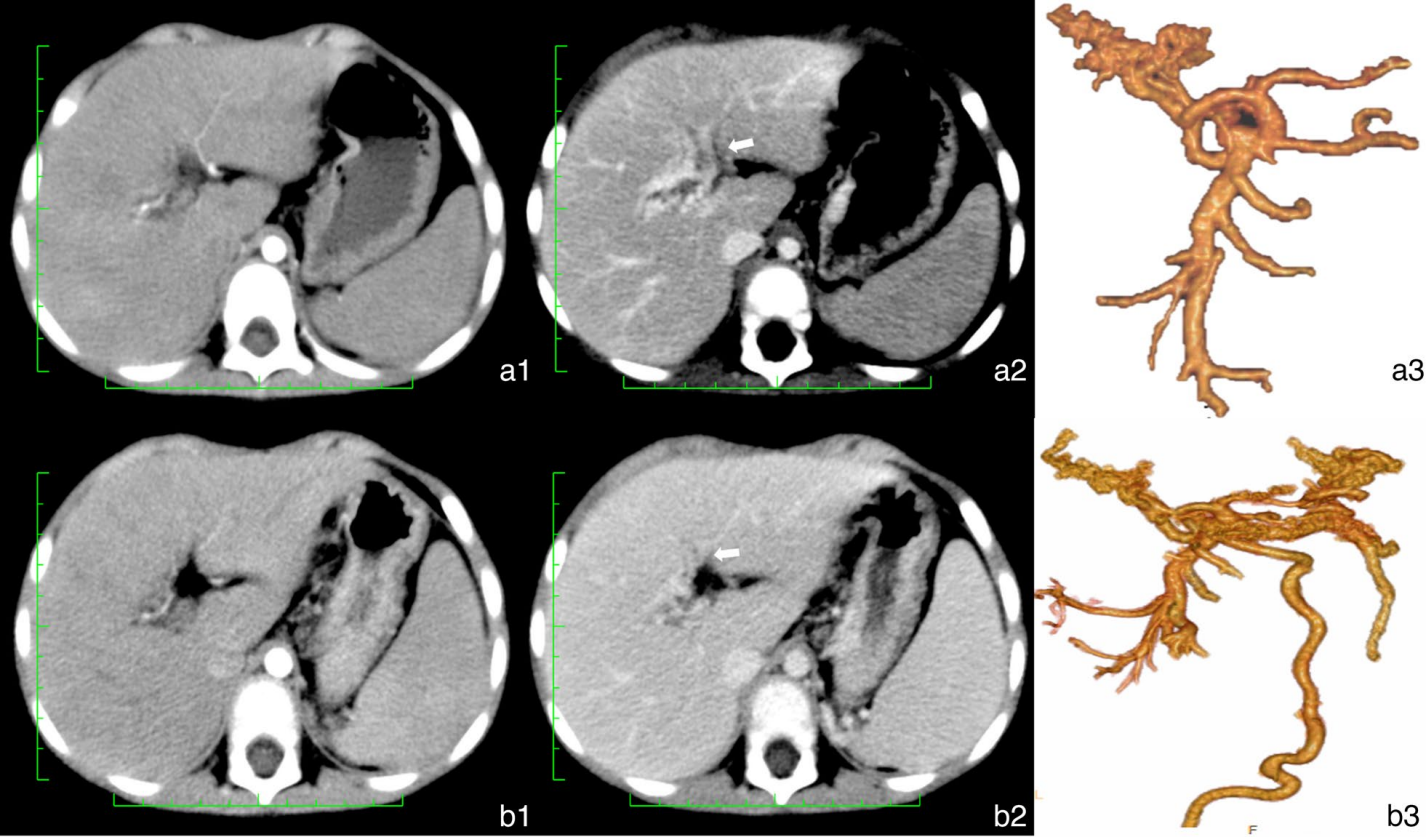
Male patient aged 2 years 6 months with a 6-month history of black stool. a The Rex recess was faintly visible and the diameter of the Rex recess (white arrow) was 1.8 mm (type 3). b Computed tomography angiography after 18 months. Complete loss of vascular landmarks of the Rex recess (white arrow), which was type 4. b3 Volume rendering showed an increase in cavernous transformation
